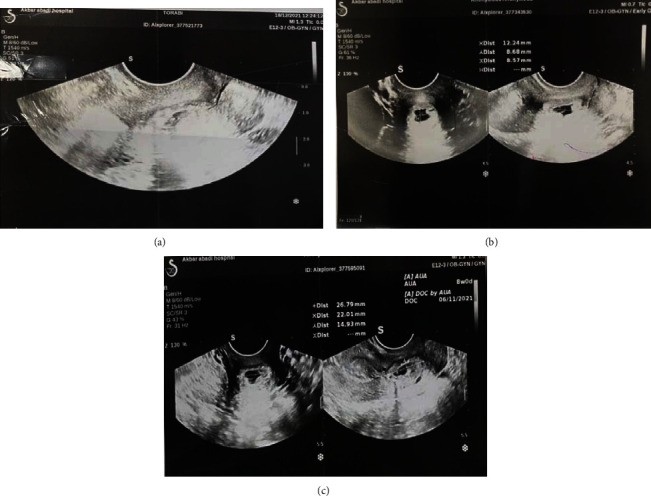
The 3^rd^ ultrasonography, a fetal echo (with FHR +) in the pregnancy bag.
